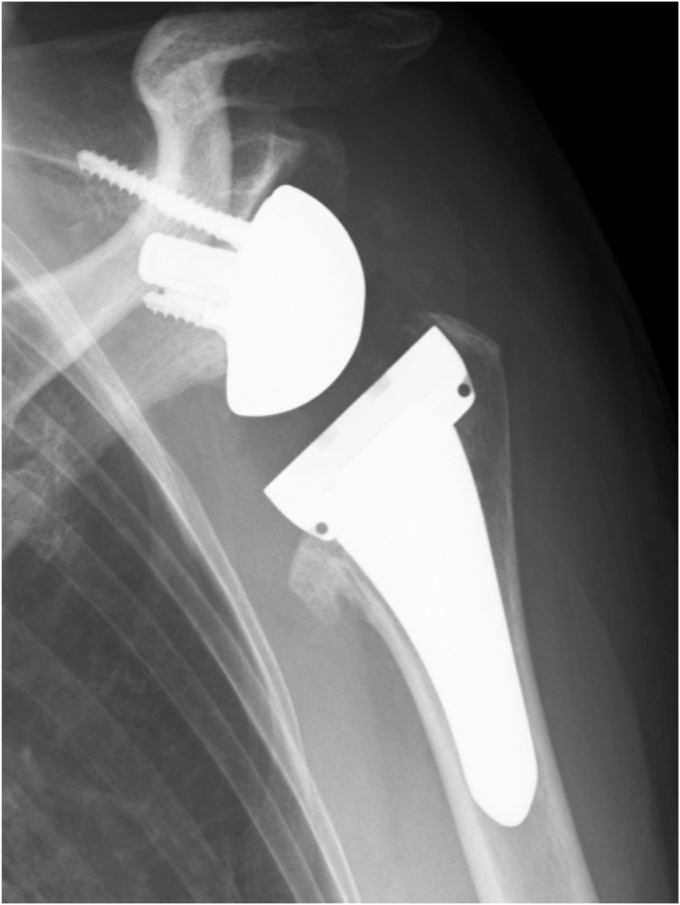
Postoperative radiograph two years after reverse shoulder arthroplasty.
